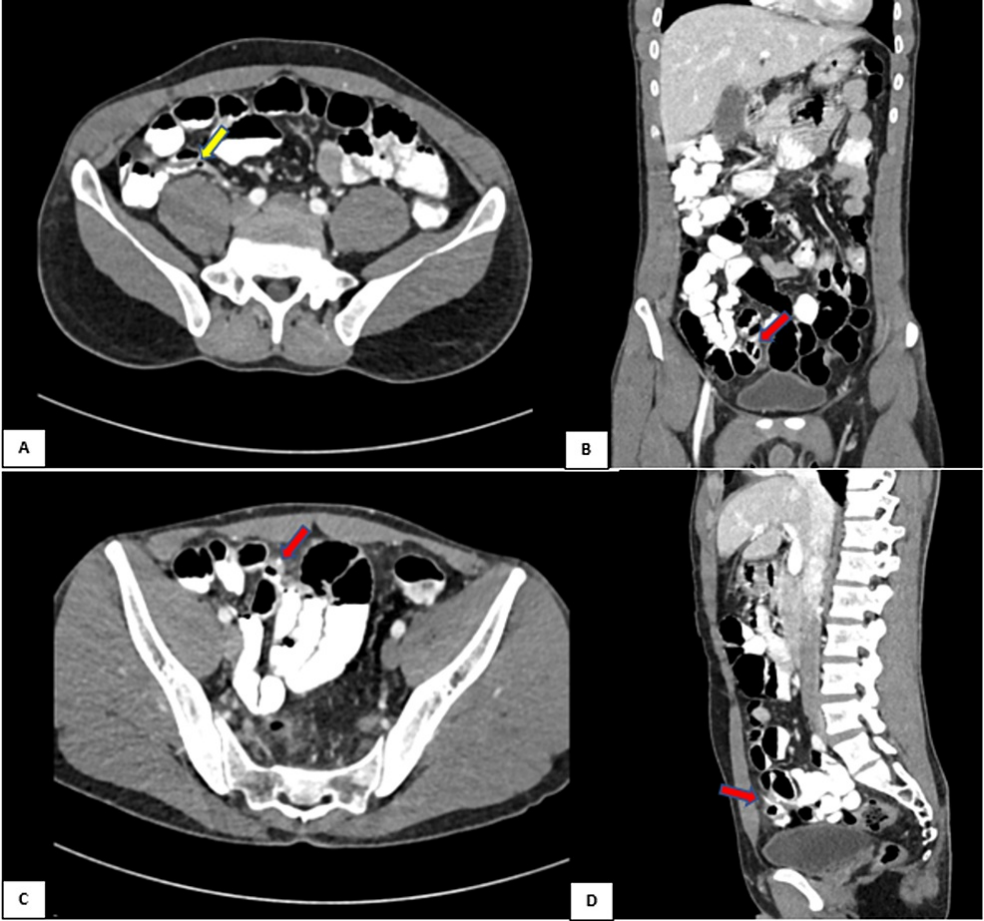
A) Axial CT abdomen and pelvis with oral and IV contrast showing appendix of normal calibre (yellow arrow) with no periappendiceal fat stranding. B) Coronal, C) Axial, and D) Sagittal cuts showing foreign body (fish bone) perforating the distal ileal loop (red arrows).
